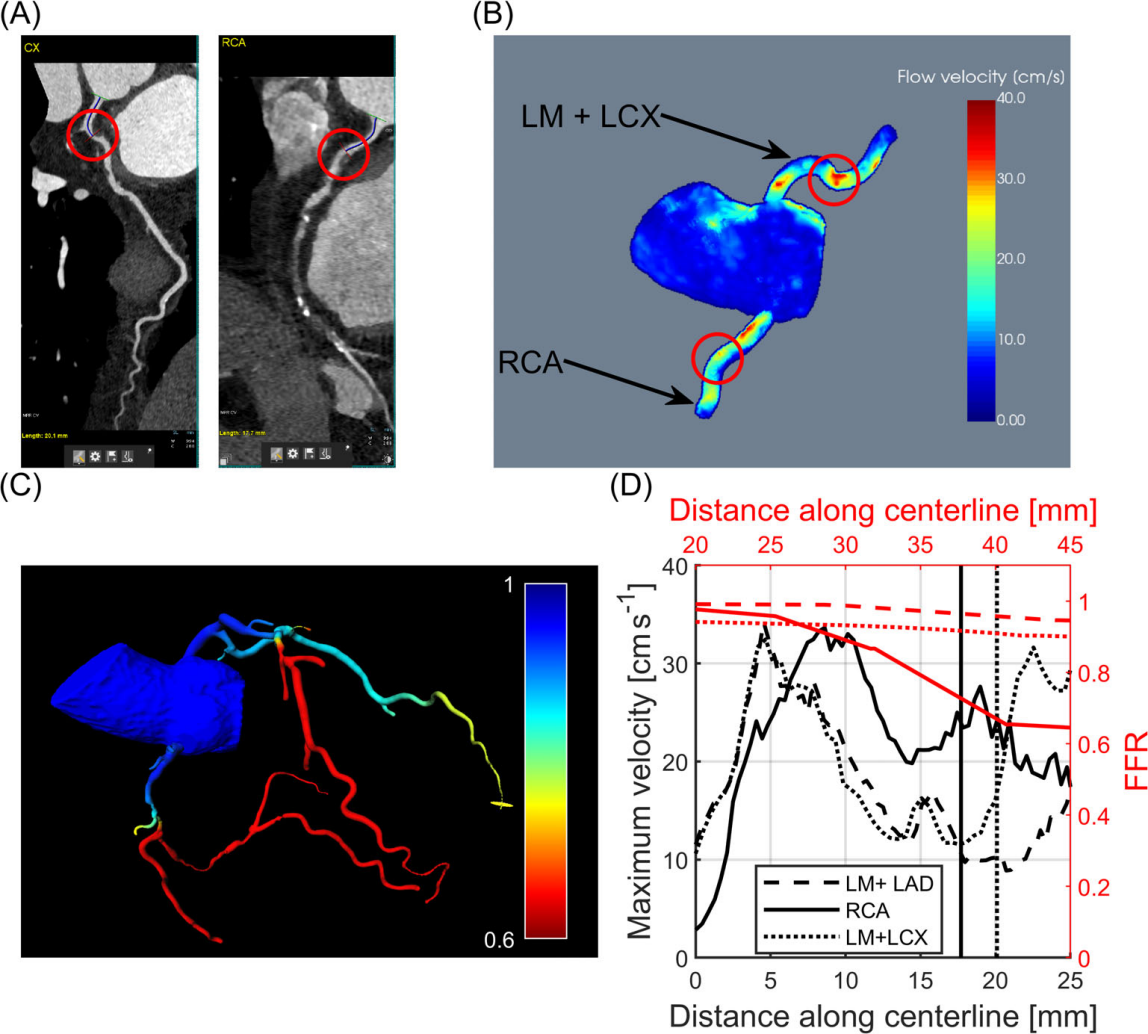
Scan results of patient 1 (male, 59 years) with stenoses in the proximal left circumflex (LCX) and right coronary artery (RCA). (A) Reformatted CT scans of the LCX and RCA. Stenoses in the proximal vessels are marked by red circles. (B) A maximum intensity projection of flow velocities acquired using our proposed 3D phase contrast (PC) sequence for the RCA and the combined left main (LM) and LCX. For display purposes, the LAD is not shown here to avoid overlap with other arteries and therefore, falsification of the apparent velocity values. (C) Distribution of fractional flow reserve (FFR) values throughout the coronary vessel tree obtained from CT scans. (D) Quantitative flow velocities corresponding to (B) as well as FFR values are plotted against distance along the vessel centerlines. The distances of the stenoses from the respective vessel ostia were measured based on the CT scans and are marked by vertical lines.
